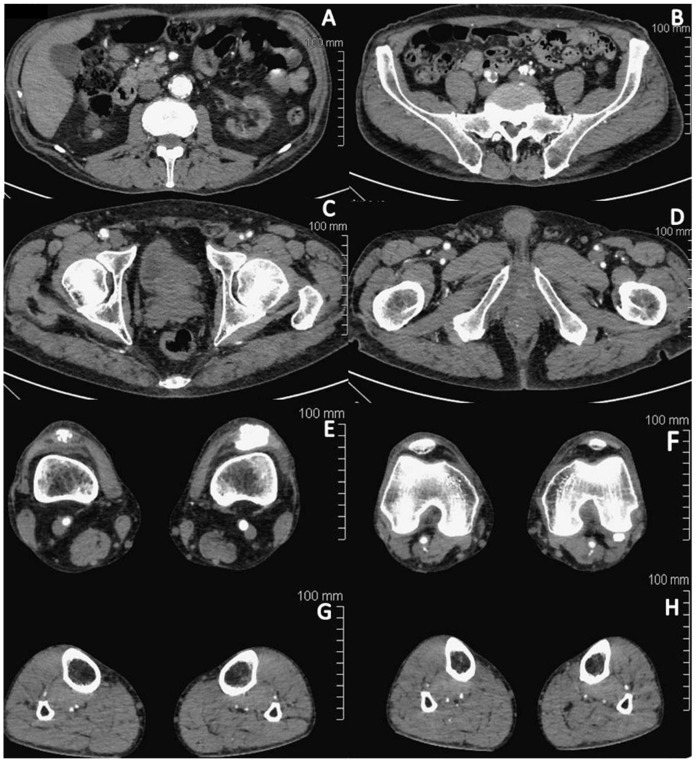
Angio-CT scan of the vascular axis in the case patient. (A) abdominal aorta with severe calcification and thrombotic plaques; (B) common iliac arteries with diffuse concentric parietal thrombotic apposition; (C) femoral arteries with diffuse parietal fibrocalcific apposition with irregular lumen; (D) femoral arteries with reduced lumen, particularly on the left side. (E) On the right side, pervious and diffusely atheromatic femoral popliteal axis. On the left, diffuse thrombotic apposition of the superficial femoral artery with presence of stenting to the popliteal axis. (F–H) Posterior tibial and interosseous arteries appear threadlike and tenuously opacified, especially in distal sections.
